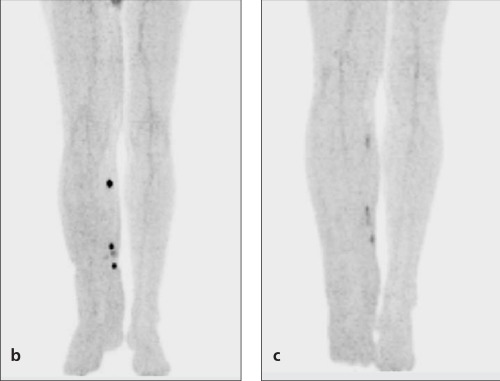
Follow up PET/CT images showed the significantdecrease in the amount of FDG uptake (1B;SUV_max_:5.2) and the firstyear examination after surgery and chemotherapy revealed favorableresponse to therapy ( 1C; SUV_max_: 2.8)
